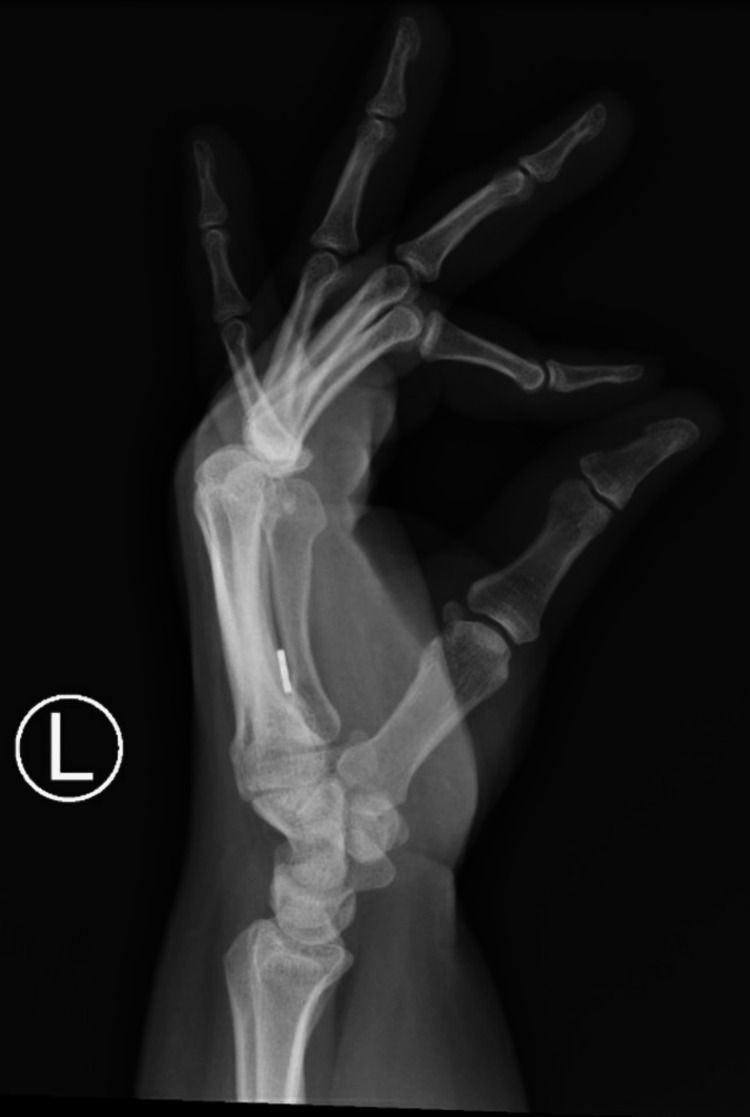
Post-operative (eight weeks) lateral radiographs.
